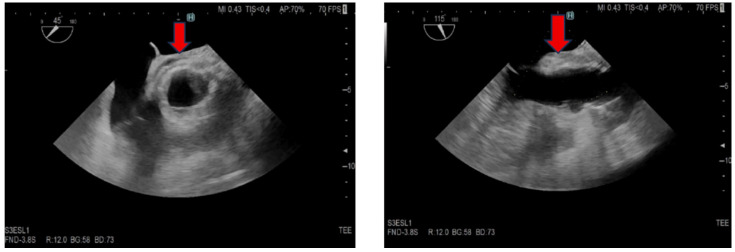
Transesophageal echocardiography, short and long axis of the aorta. The red arrow indicates thickened tissue in the aortic root area, suggestive of an abscess.
